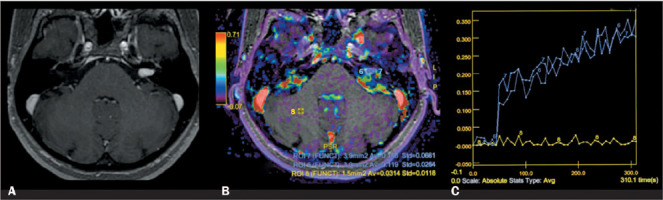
MRI scans of a 64-year-old female with a left-sided Hannover T2 VS. A: Axial
